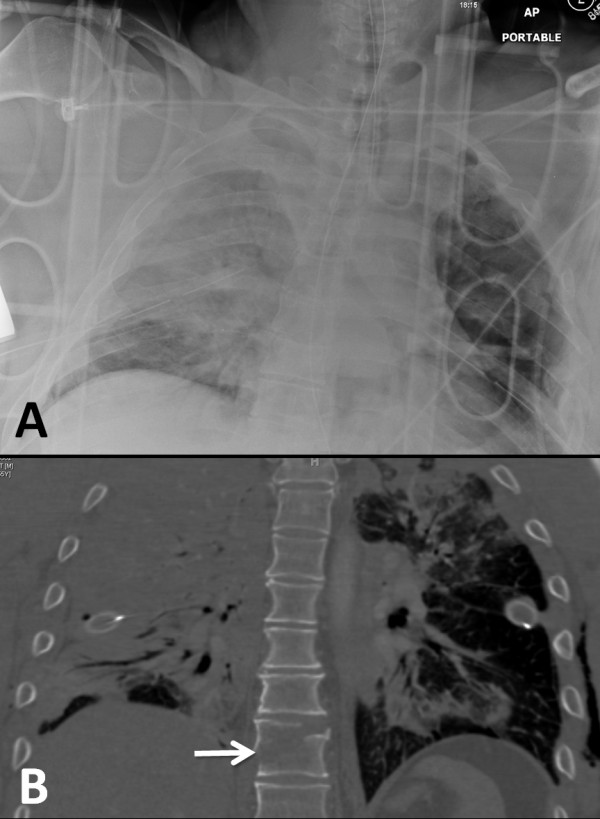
Initial chest radiograph (A) and coronal CT scan reconstruction (B) on arrival in the emergency department. Despite placement of bilateral chest drains, there is a persistent, extensive hemothorax on the right side, and signs of bilateral lung contusions. The arrow in panel B points out the T9 hyperextension injury in the coronal plane.
